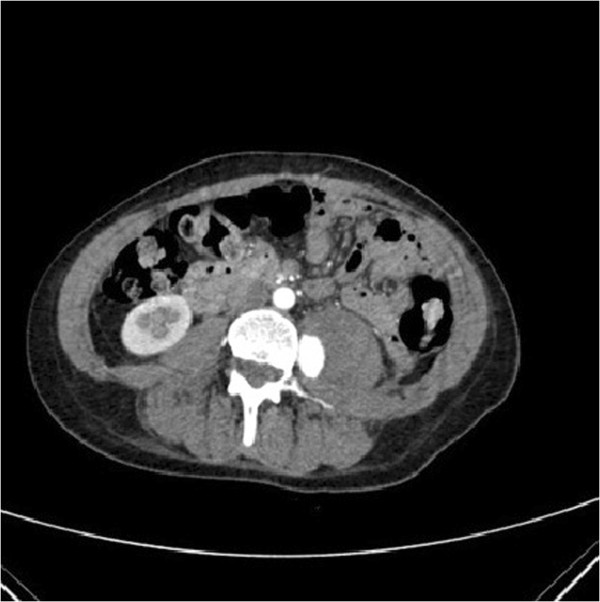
CT scan image during the excretory phase, which show a left psoas iliac muscle haematoma with an image consistent with a pseudoaneurysm.
